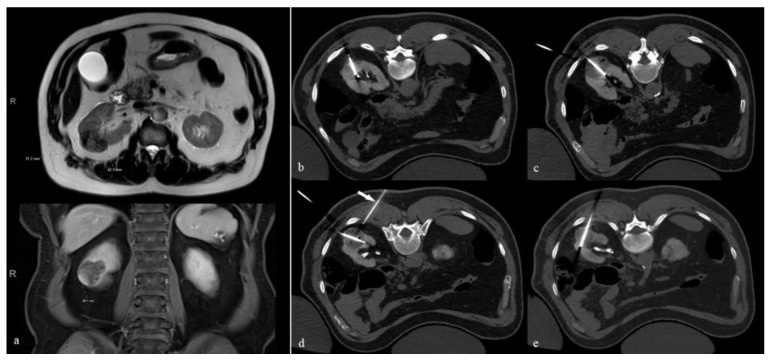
Cryoablation of a right renal RCC. (a) MRI scan shows a right renal partly exophytic mass, measuring 4.2 × 3.1 × 4.5 cm along the axial and craniocaudal dimensions, respectively. (b–e) Four cryoablation probes are carefully aligned along the longitudinal axis of the mass from cranial to caudal. In our experience, the orientation of the probe shown in figure (e) is critical as it allows the ice ball to ablate the most central part of the mass, which is a common site for recurrence. The arrow in (d) points to a 21 G needle used for CO_2_ dissection, as shown in Figure 2.
